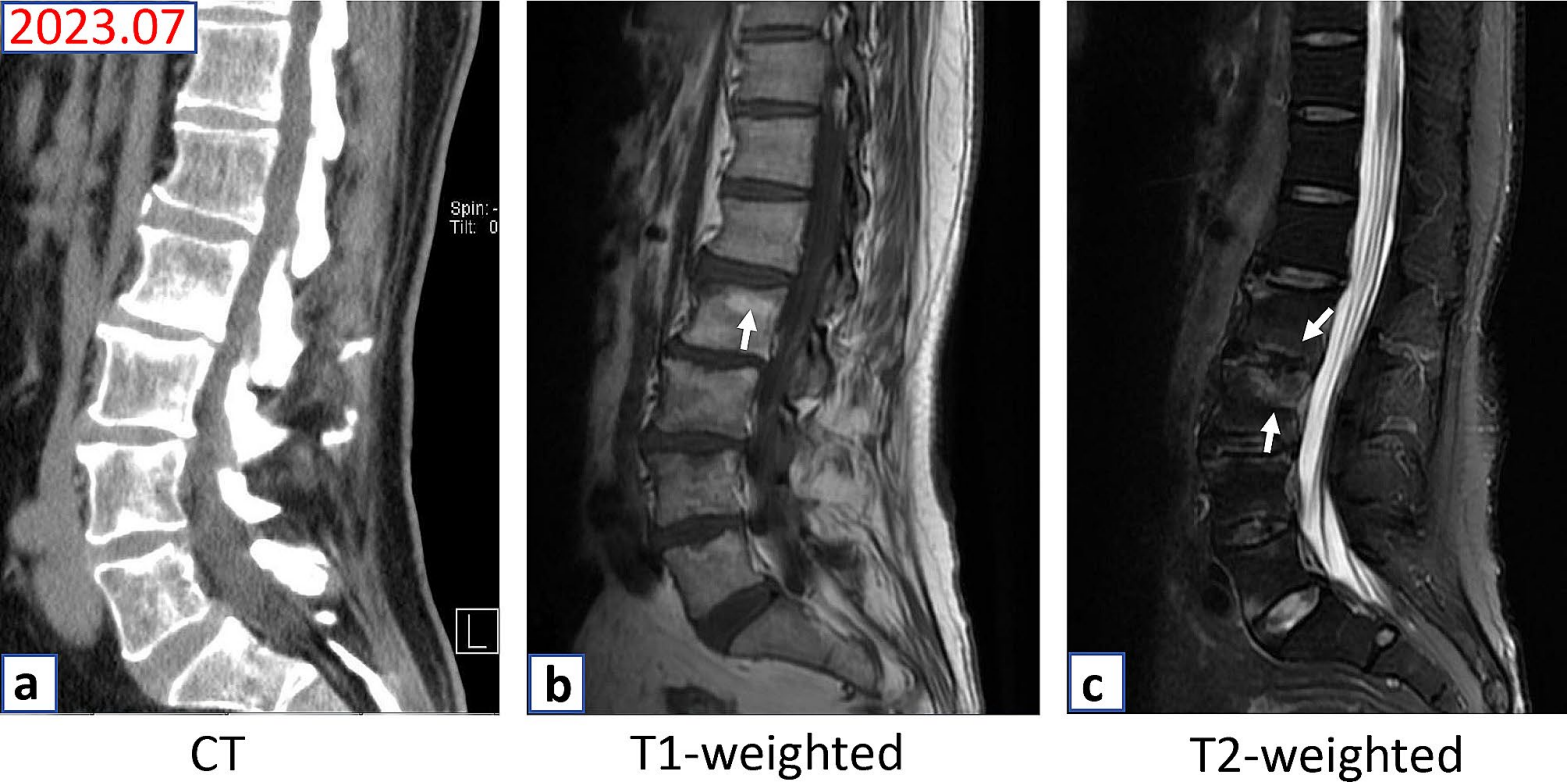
Imaging manifestations after treated with anti-fungal therapy. CT showed an significant reduction of bone destruction in vertebral body (a). MRI showed reduced bone destruction at the L2 and L3 vertebral body (white arrow) compared with the imaging performance on July, 2022 (b&c)
